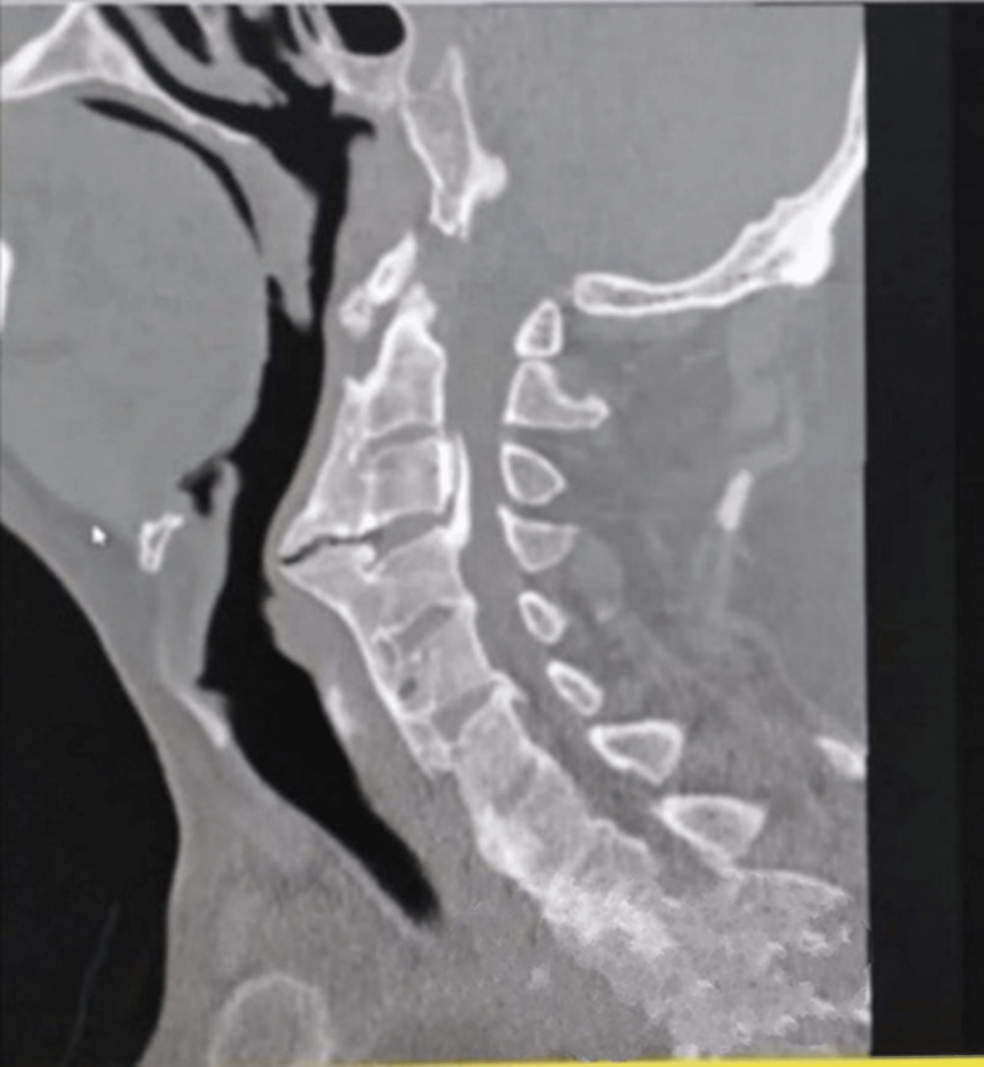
Cervical spine CT without contrast showed that the osteophyte on the anterior surface of the spine protruded and constricted the esophagus.
